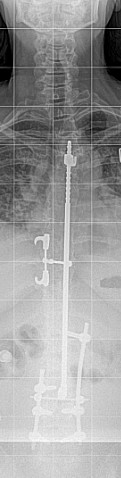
X-ray of the spine in anterior-ppsterior projection after dorsal spondylodesis
